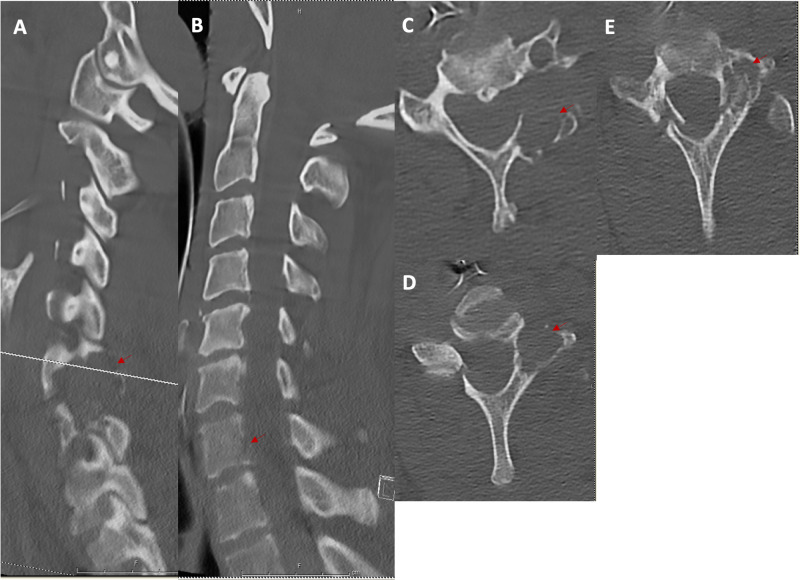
Cervical CT of traumatic fracture and osteolytic lesion (A) Left parasagittal cervical CT demonstrating osteolytic lesion (red arrows) at cervical 6 and 7 levels. (B) Mid-sagittal cervical CT cervical 7 vertebral body fracture without compression of the spinal cord. (C) Axial CT at the level of cervical 6 demonstrating osteolytic lesion originating at the left facet joint and abutting the left vertebral foramen. (D) Axial CT at the level of cervical 7 demonstrating an osteolytic lesion originating at the left facet joint with visualization of right laminar fracture and partial visualization of left laminar fracture. (E) Axial CT at the level of cervical 7 better demonstrating left laminar fracture CT: computed tomography
